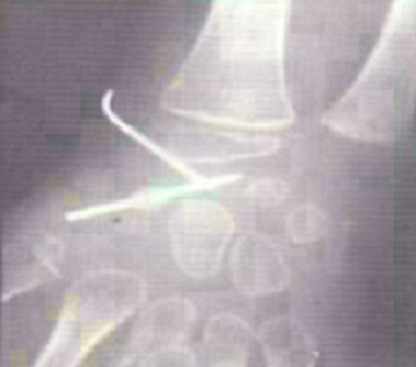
Aspect radiographique de face post opératoire après réduction chirurgicale, stabilisée par des broches radio et scaphlunaire
